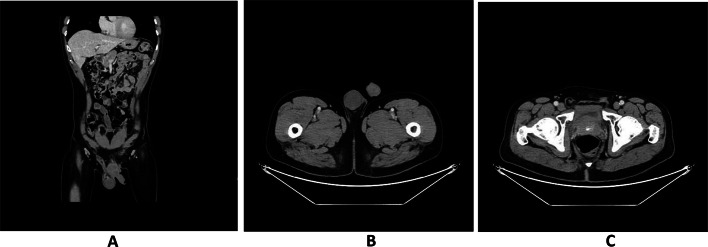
Follow-up CT examination; Absence of left epididymal and testicular (A, B). Surgical scar at the left inguinal region (C)
